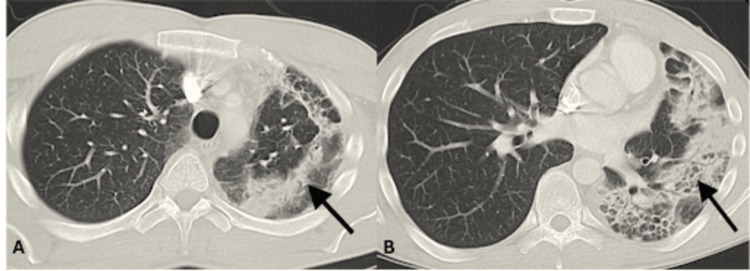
CTA done on 6/10/2022 showing left peripheral parenchymal infiltration (black arrow). CTA: computed tomography angiography
